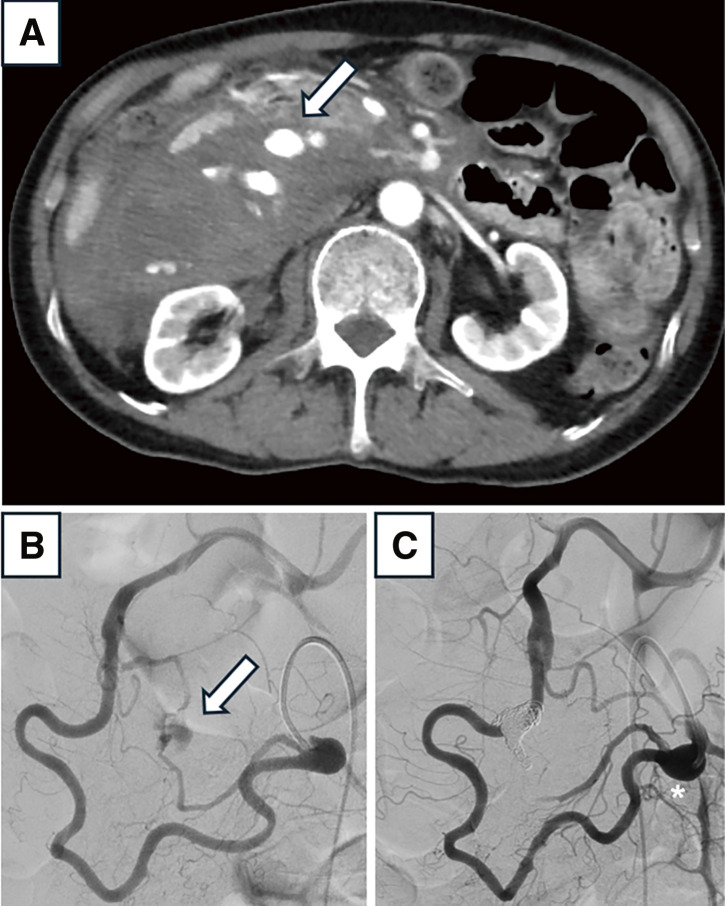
Fig. 1 (A) Pre-TAE axial computed tomography angiography showing ruptured SPDAA (arrow) and large intra-abdominal hematoma. (B) Angiography via the superior mesenteric artery revealing ruptured SPDAA (arrow). (C) Post-TAE angiography showing occlusion of the SPDAA and residual inferior pancreaticoduodenal artery aneurysm (*). SPDAA: superior pancreaticoduodenal artery aneurysm; TAE: transcatheter arterial embolization
